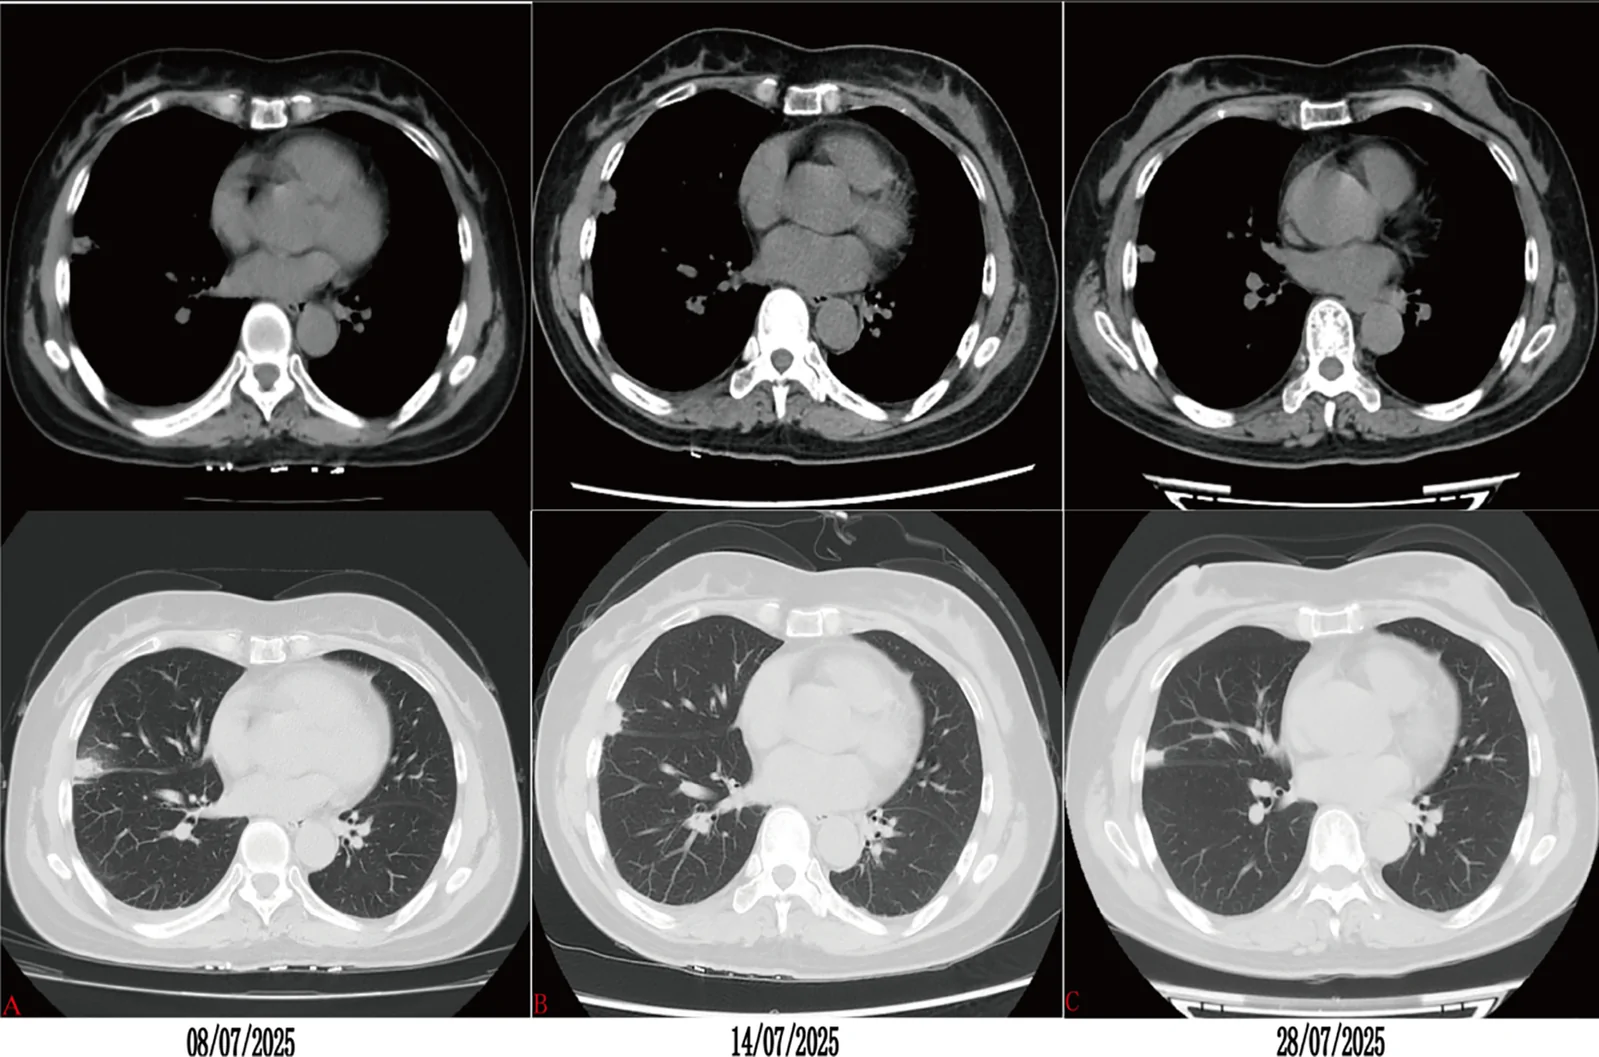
A, B, and C represent the results of 3 pulmonary CT examinations conducted in July 2025 on a patient who underwent mixed hemorrhoid surgery in this case. CT = computed tomography.
